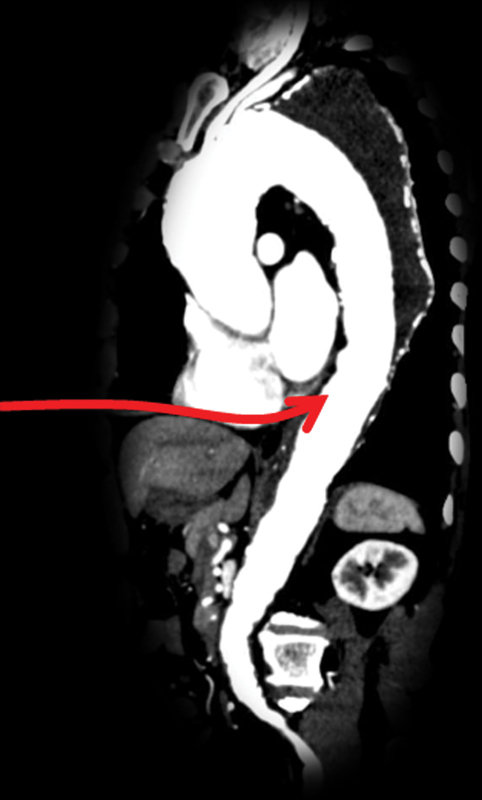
Sagittal section of computed tomography showing the proximity of the distal descending thoracic aorta to the heart.
